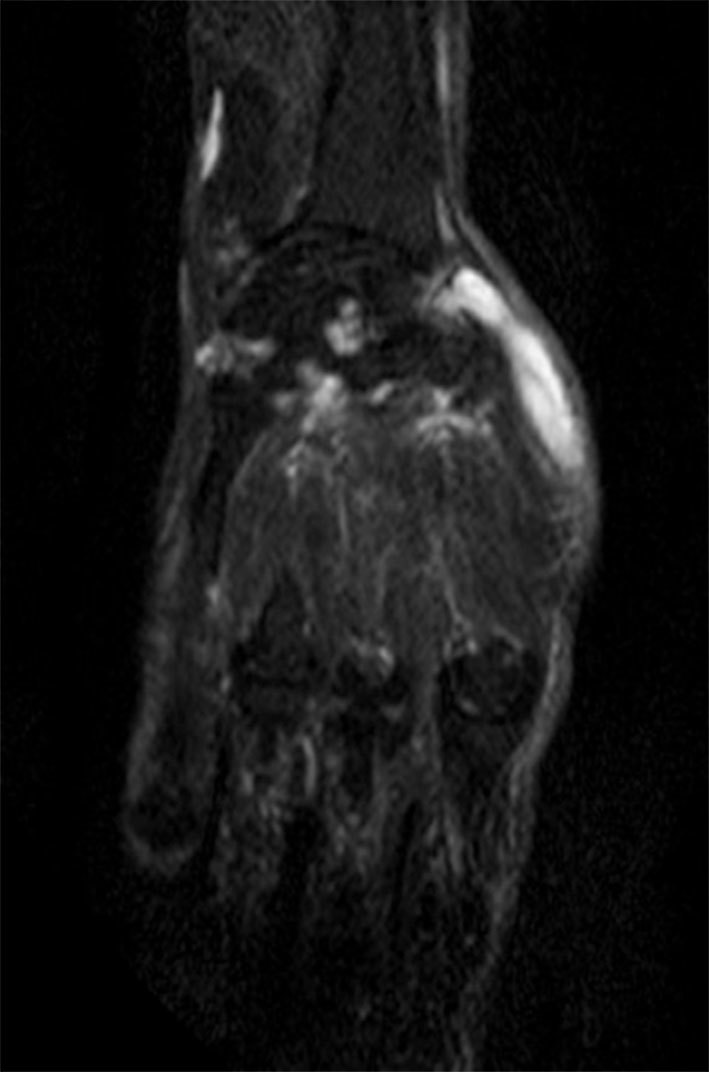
Short tau inversion-recovery MRI showing a cystic lesion involving the lateral aspect of the wrist (along the abductor pollicis longus and the extensor pollicis brevis).
